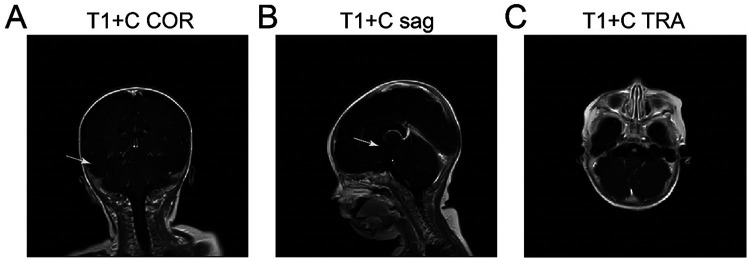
Contrast-enhanced brain MRI on May 16, 2025. (A) Coronal T1-weighted postcontrast image. (B) Sagittal T1-weighted postcontrast image. (C) Axial T1-weighted postcontrast image.
